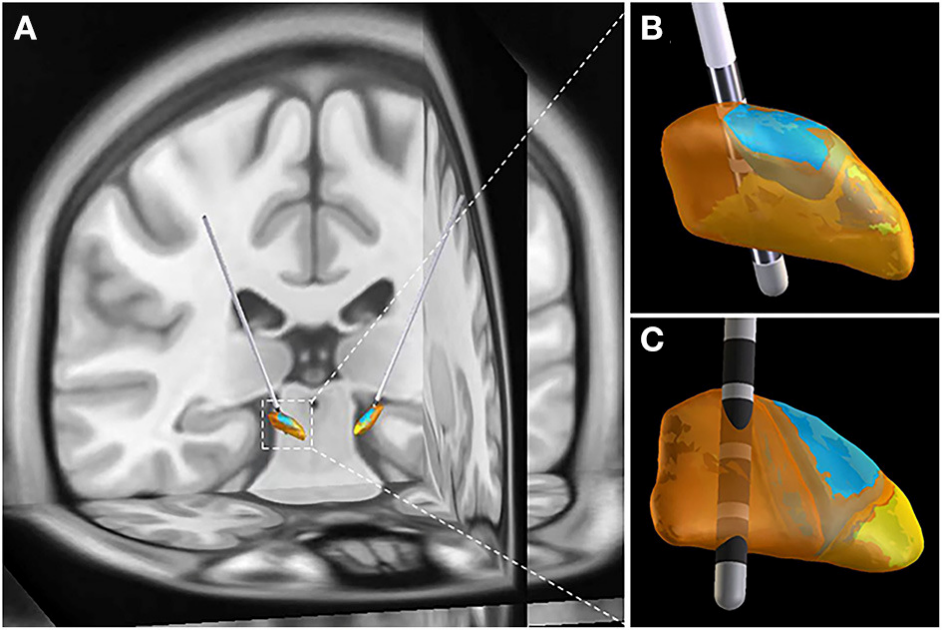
Representative case with electrodes implanted into sensorimotor STN. (A) 3-D image reconstructed by preoperative MRI image and postoperative CT image. (B) Anterior view of STN. (C) Posterior view of STN. STN is partitioned into sensorimotor (orange), limbic (yellow) and associative (blue) subregions. The image reconstruction was performed by Lead-DBS.
